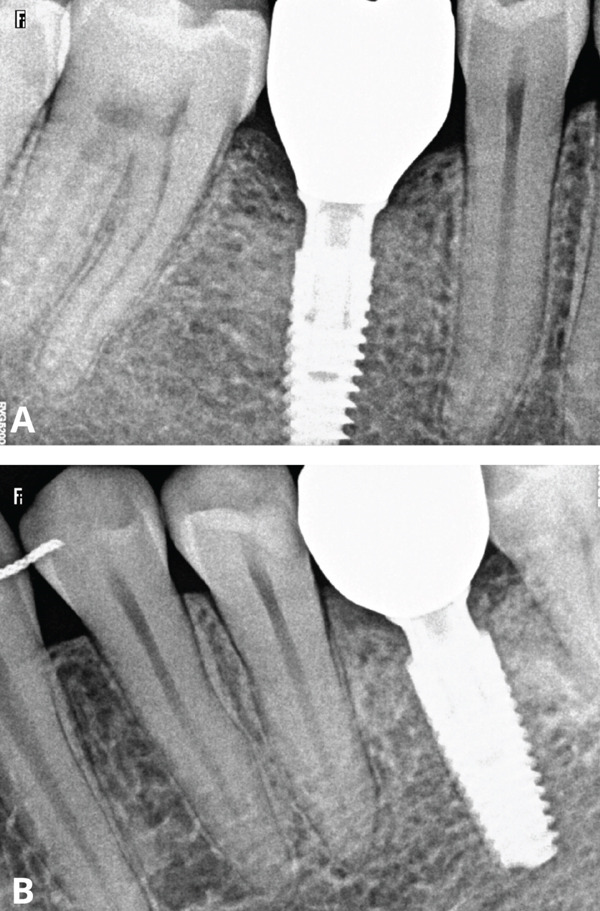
Follow‐up radiographs of implants after 3 years. (A) Site 36 and (B) Site 46.
